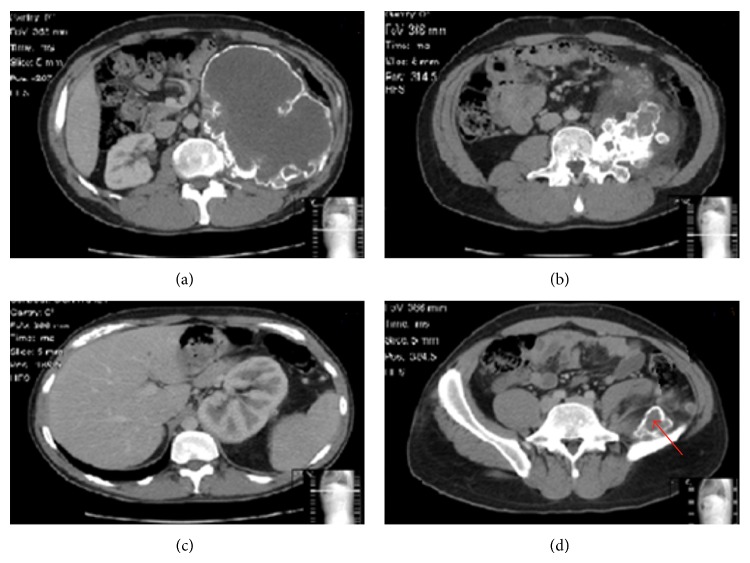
CT scan (a) axial view at L3 showing the distinct peripheral calcification of this large lesion and the displacement of intra-abdominal organs and blood vessels. (b) Axial cut through the middle third of the L3 vertebra depicting the L3 vertebral pedicle origin of the lesion with marked intralesional calcification. (c) Axial view at the level of T12 showing the superior displacement of the left kidney. (d) Axial view at the level of S1 showing the lesions inferior extension into the pelvis (red arrow).
